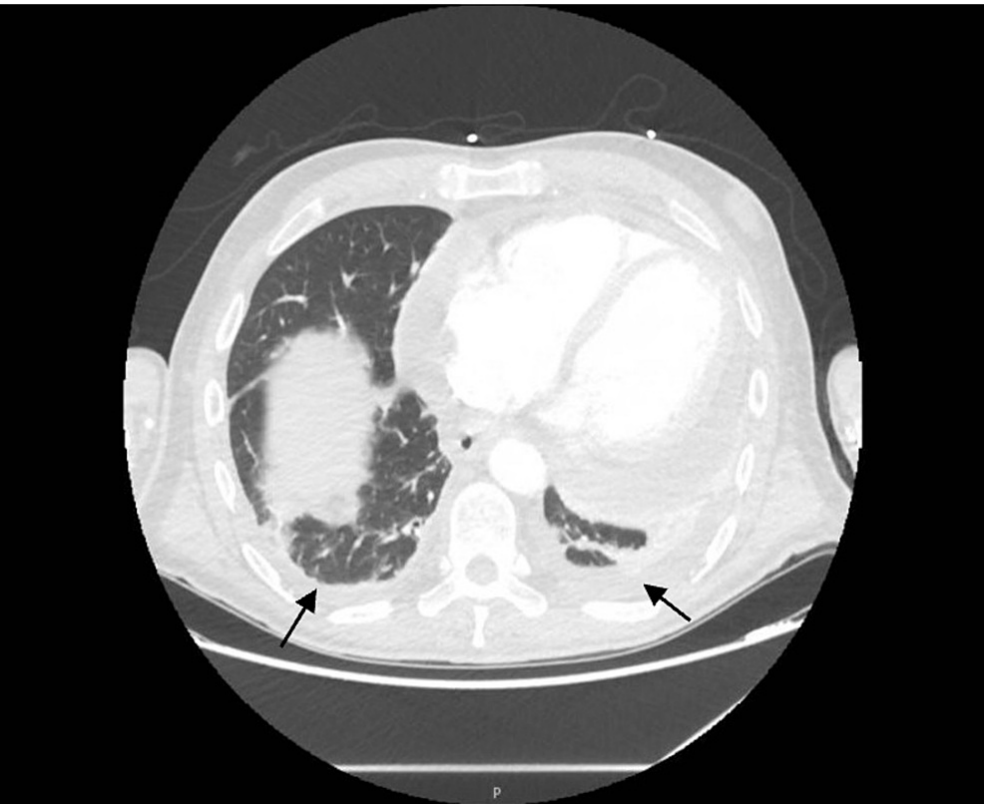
CTA chest (black arrows are pointing to large pericardial effusion) CTA: computed tomography angiography
